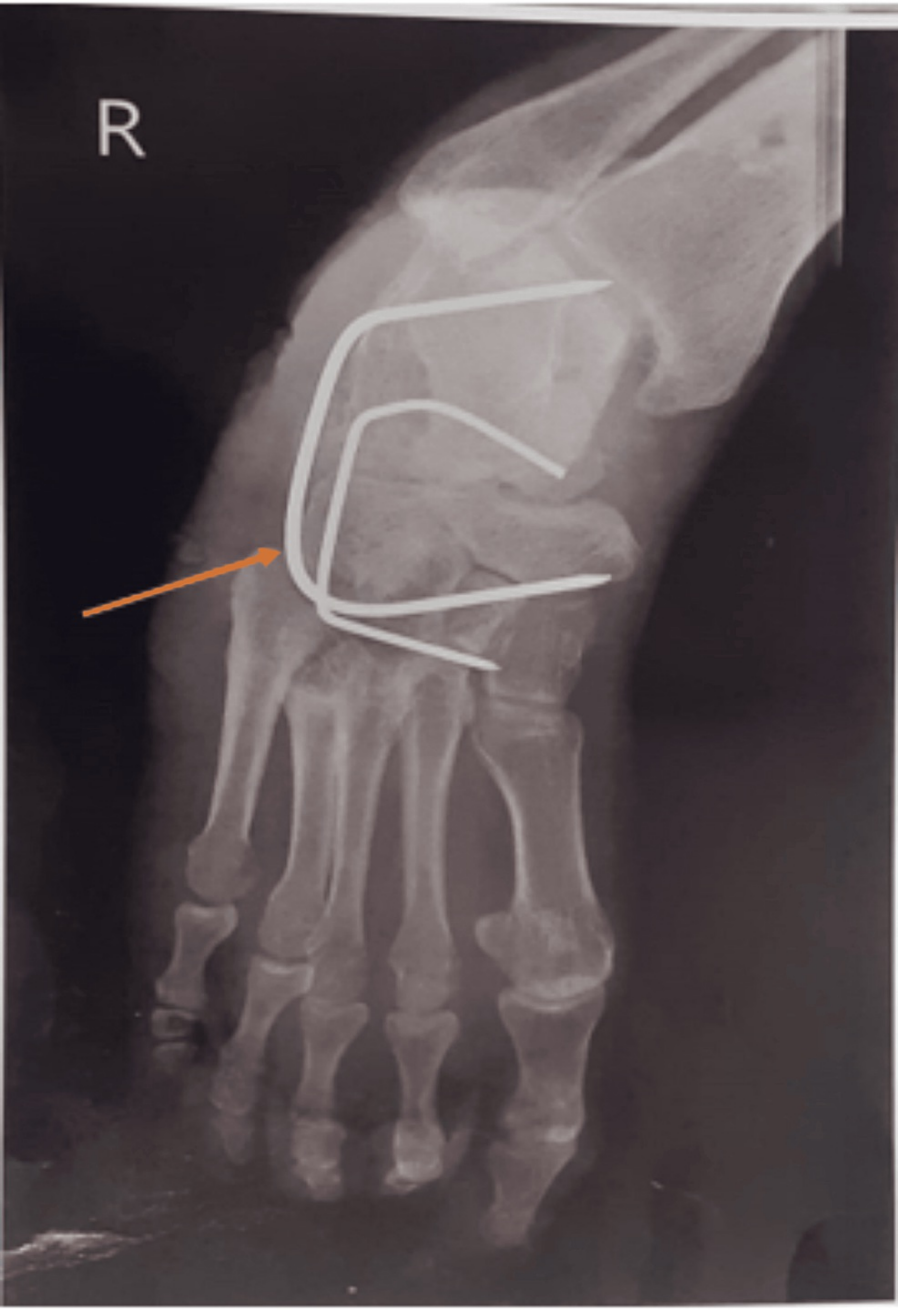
Post-operative X-ray of Charcot's foot with equinovarus deformity with k-wire.
